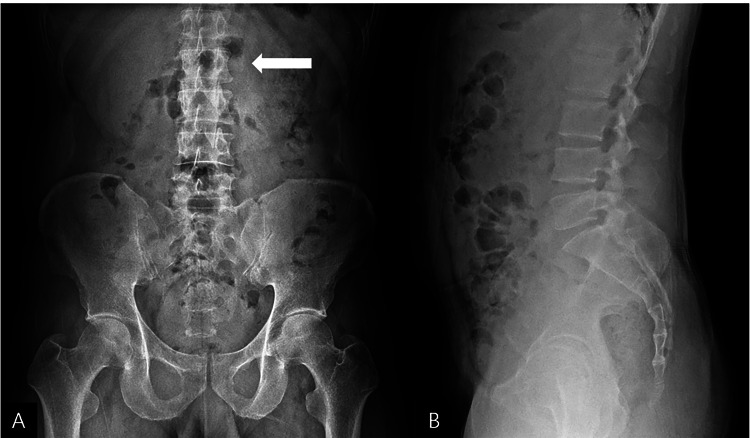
X-ray imaging of lumbar spine with identified fracture Lumbar spine X-ray. (A) AP View: No definite fracture line is present; however, subtle cortical irregularities at the tip of the left L2 transverse process are noted (arrow). (B) Lateral View: No significant vertebral body fracture or degenerative changes are observed.
